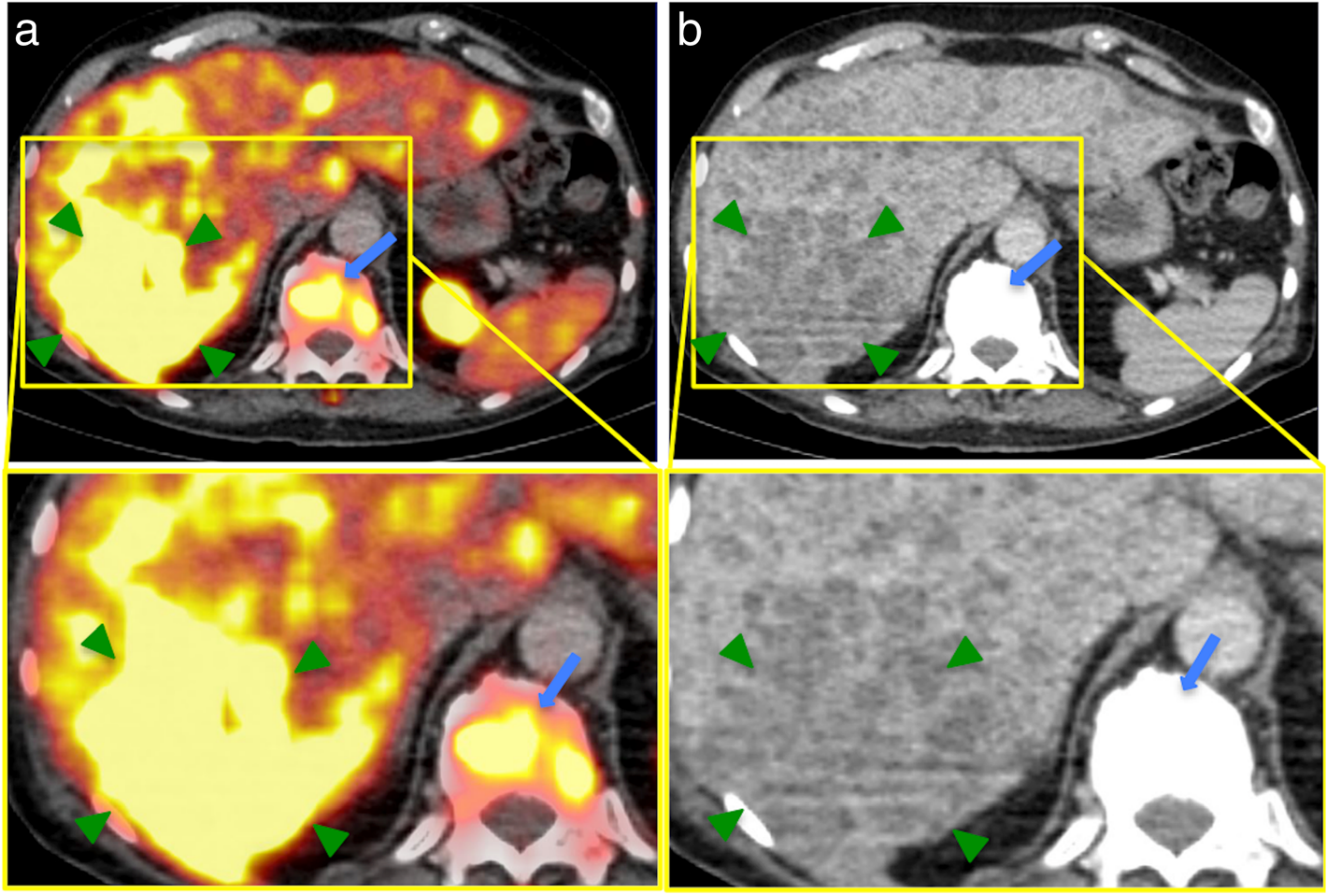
Example of ^68^Ga-PSMA-positive liver metastases in a PC patient with a recurrent acinar adenocarcinoma. a, b: ^68^Ga-PSMA-PET/CT of a 68-year-old patient with a recurrent acinar adenocarcinoma of the prostate. At initial diagnosis in 2007, the GS was 5 + 5. The patient received primary radiotherapy and undergone chemotherapy as well as androgen-deprivation therapy. The serum PSA was 606 ng/ml at the time of examination. Besides disseminated osseous metastases (such as in a vertebral body, blue arrows) and a singular lymph node metastasis in the axilla, the PET/CT (a) revealed small-nodular, PSMA-positive liver metastases in all segments, with SUV_max_-values up to 26.3 (exemplary in segments VII/VIII, green arrows). In contrast-enhanced CT (b), liver metastases appear ill-defined and hypodense compared to the liver, typical for hypovascular metastases. *GS* Gleason score, *PSA* prostate-specific antigen, *SUV*_*max*_ Maximum standardized uptake value
